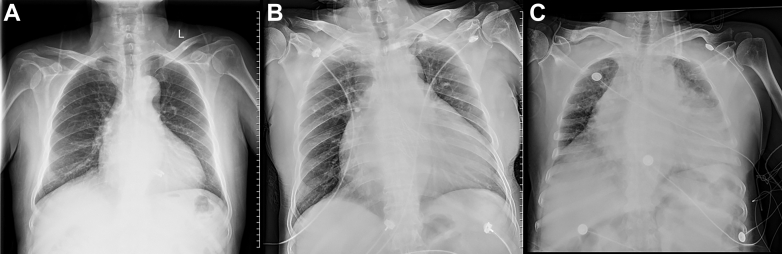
Evolution of Chest Radiography From First Admission to Recovery (A) Chest radiograph showed that the heart/chest ratio was 0.63 at discharge and recovery. (B) The heart/chest ratio was 0.81 at the second admission to the cardiac care unit. (C) The heart/chest ratio was 0.9 at the first admission to the cardiac care unit with cardiogenic shock and severe pulmonary edema.
